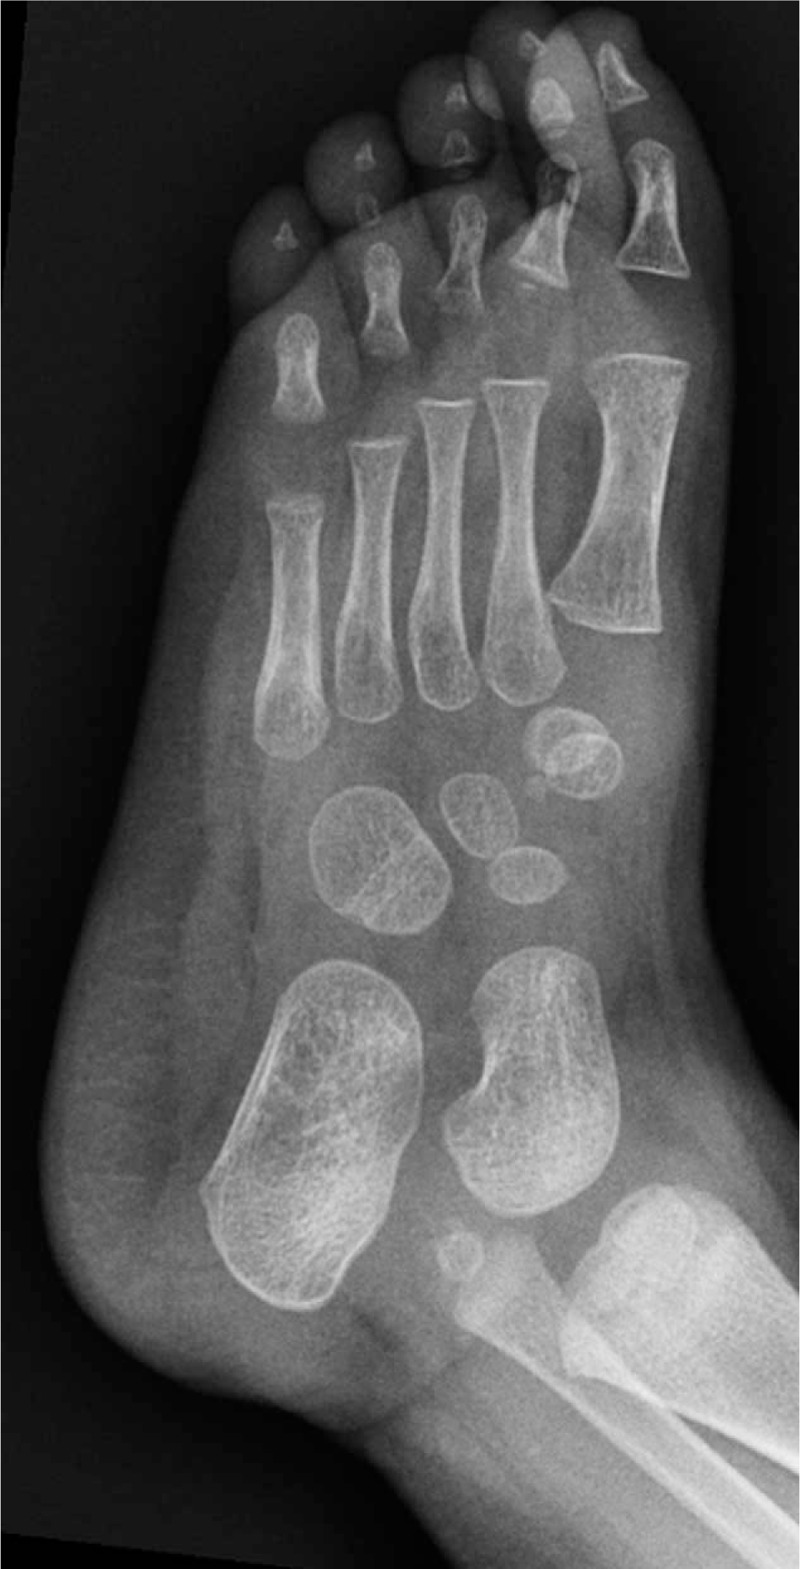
X-ray of final result (oblique plane).
